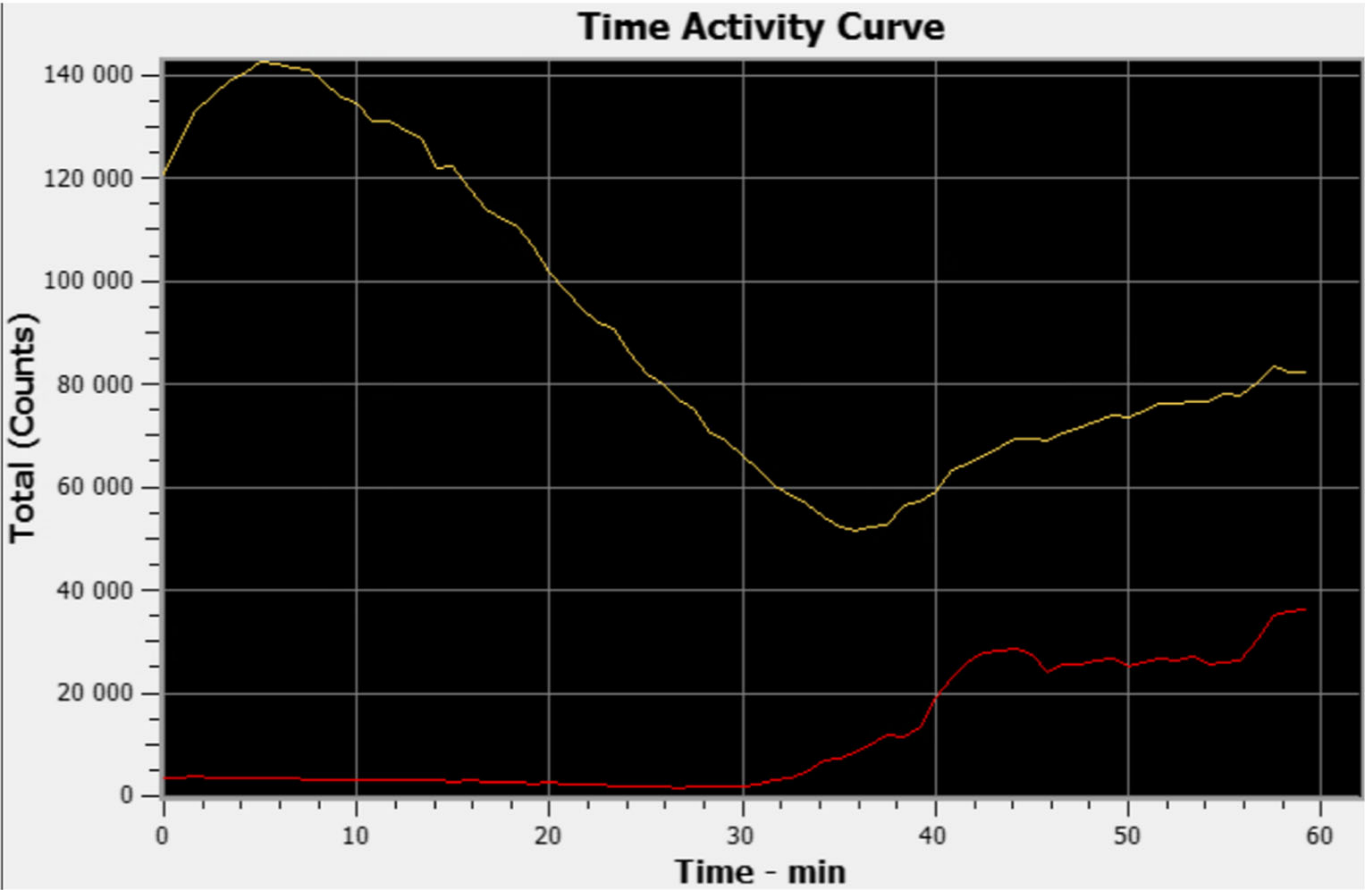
Time activity curve of the dynamic series of a bile reflux scintigraphy. Bile tracer activity in the liver (yellow line) and in the gastric pouch (red line) of one representative patient is shown. A subsequent rise in the tracer activity is seen in the gastric tube and in the liver. This is most likely due to reuptake of bile acids through enterohepatic circulation
